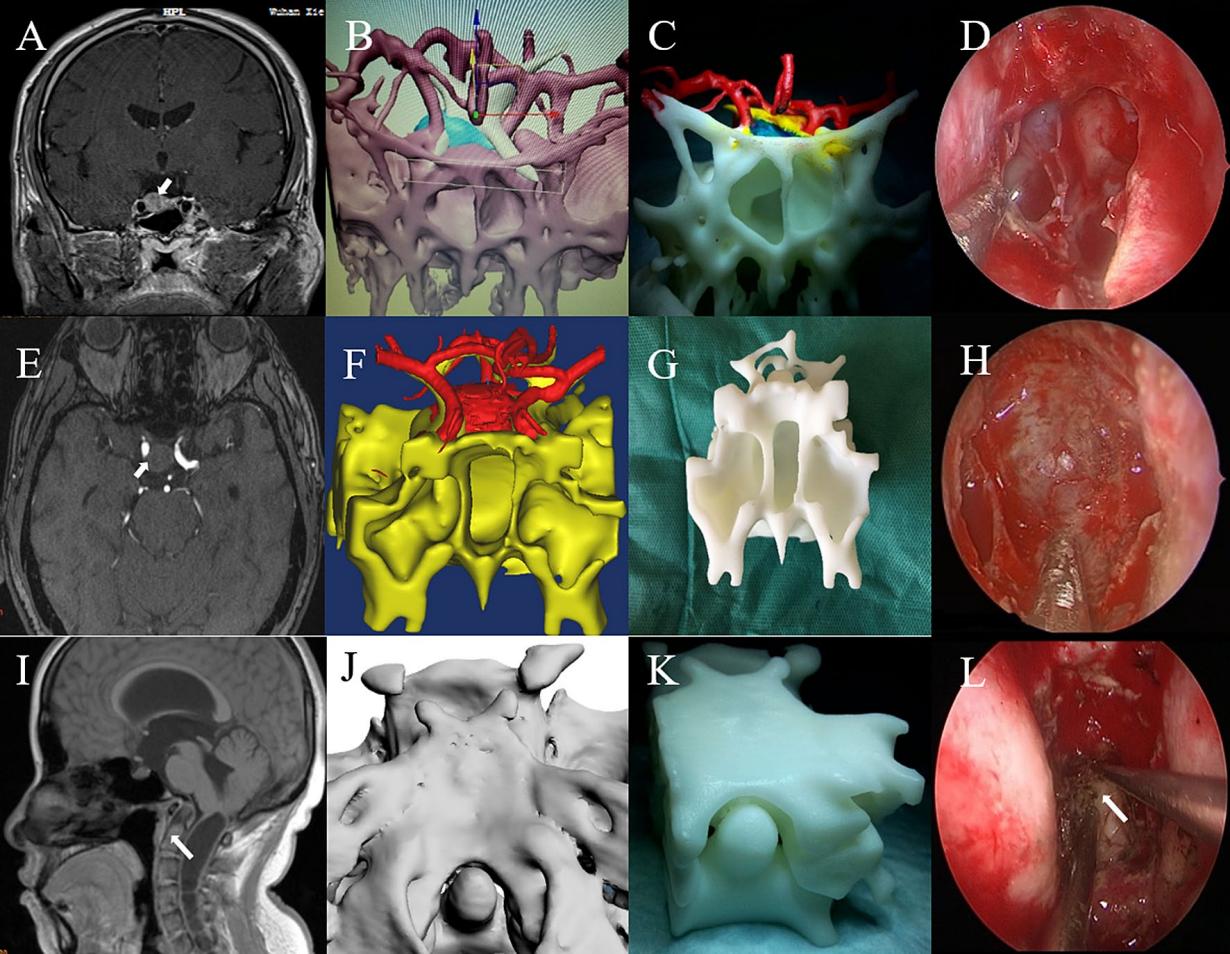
MRI, 3D printing data, and photographs of three patients. (A/E/I) MRI images of two patients with pituitary and one with odontoid deformity. The nidus is marked by a white arrow. (B/F/J) 3D reconstruction of each nidus in the software of MIMICS. (C/G/K) 3D printed models for each patient. (D/H/L) Intraoperative photographs of each patient.
